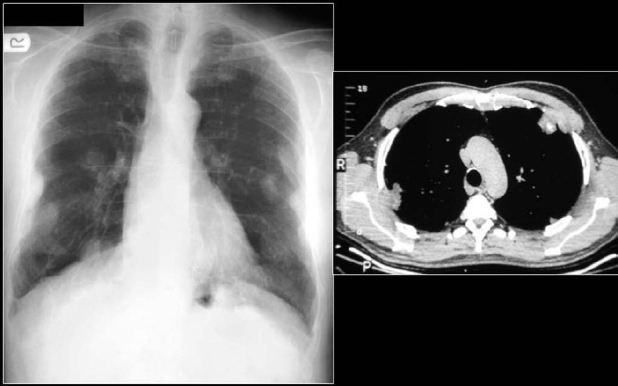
Multiple calcified pleural plaques mimicking PNs on a chest radiographs elegantly depicted on axial CT scan as calcified pleural plaques from previous asbestos exposure
